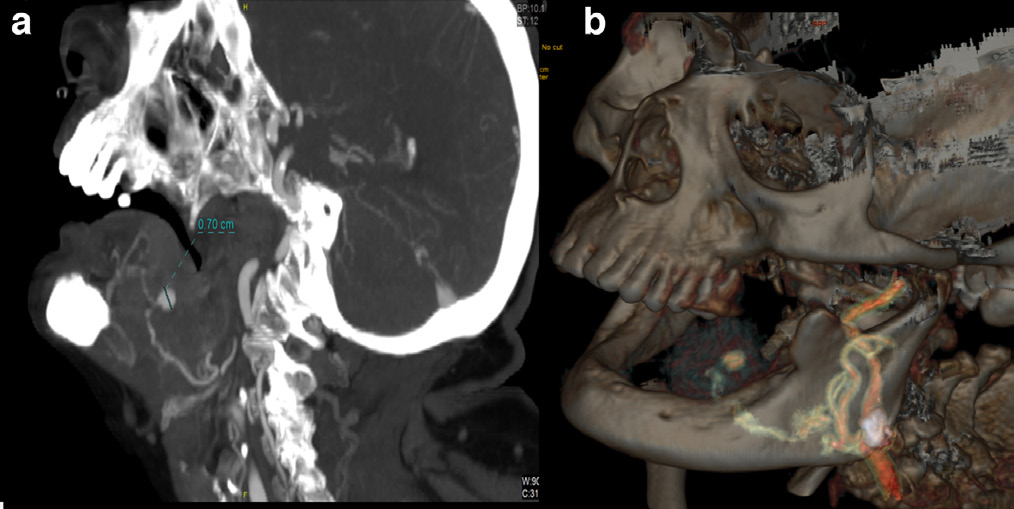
Sagittal MIP CTA (A) and 3D volume rendering (B) images depict a 7 mm aneurysmatic formation emerging from the lingual artery.
